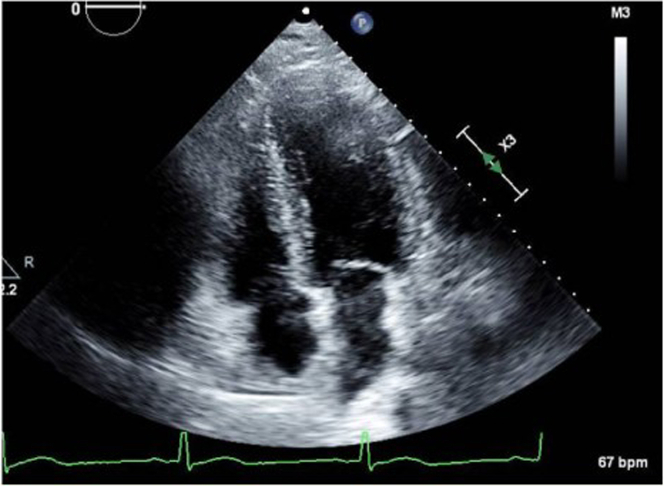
Transthoracic Echocardiography (Apical 4-Chamber View) Echocardiography demonstrating normal left ventricular size and systolic function, preserved right ventricular function, and no significant valvular stenosis or regurgitation.
